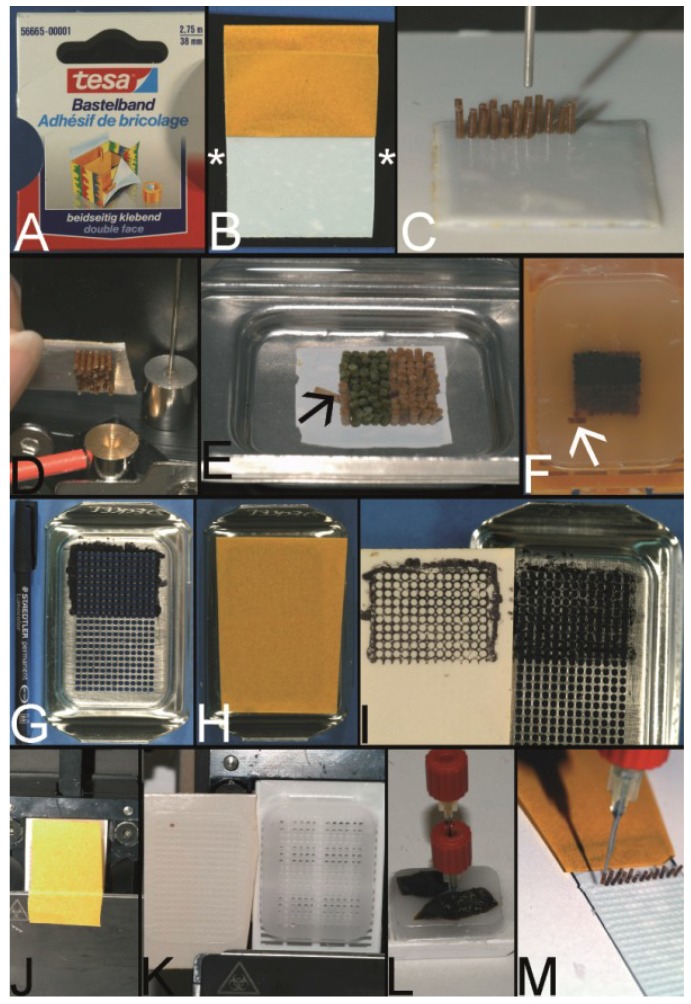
Wilkens and Chen technique—double sided adhesive tape to fix the PTCBs without the need for a preformed recipient block. (A) Example for a double-sided adhesive tape to fix the paraffin tissue core biopsies (PTCBs). (B) White double-sided adhesive tape with a brown protection paper mounted on a standard black x-ray film (asterisk). (C) PTCBs arranged in a Cartesian coordinate system on the double-sided adhesive tape. (D) Strong contact between the PTCBs and the double-sided adhesive tape—no PTCB falls off. (E) Melting of the PCTB-adhesive tape-x-ray-film-sandwich in a standard steel embedding mold. Do not heat over 65 °C, otherwise the adhesive tape may shrink and destroy the PTMA. Note that one PTCB toppled down (arrow). Not paraffin, but the paraffinized tissue must be in contact with the adhesive tape. (F) PTMA after resolidification and removal of the adhesive tape-x-ray-sandwich. Note the tumbled PTCB (arrow). (G) Routinely used steel embedding mold with many holes (see also Figure 6A) in the bottom is painted with a standard permanent marker. (H) The double-sided adhesive tape is fixed to the painted bottom of the mold. (I) After removing the tape from the bottom of the mold the grid of the mold is transferred to the tape and can be used to structure the PTMA. (J) The double-sided adhesive tape is fixed to a recipient block with preformed holes, which is mounted on a microtome clamp. (K) After cutting a 5–10 µm thick section of the preformed recipient block is fixed to the adhesive tape. (L,M) PTCBs can now be transferred manually from a paraffin donor block to the grid of the double-sided adhesive tape.
